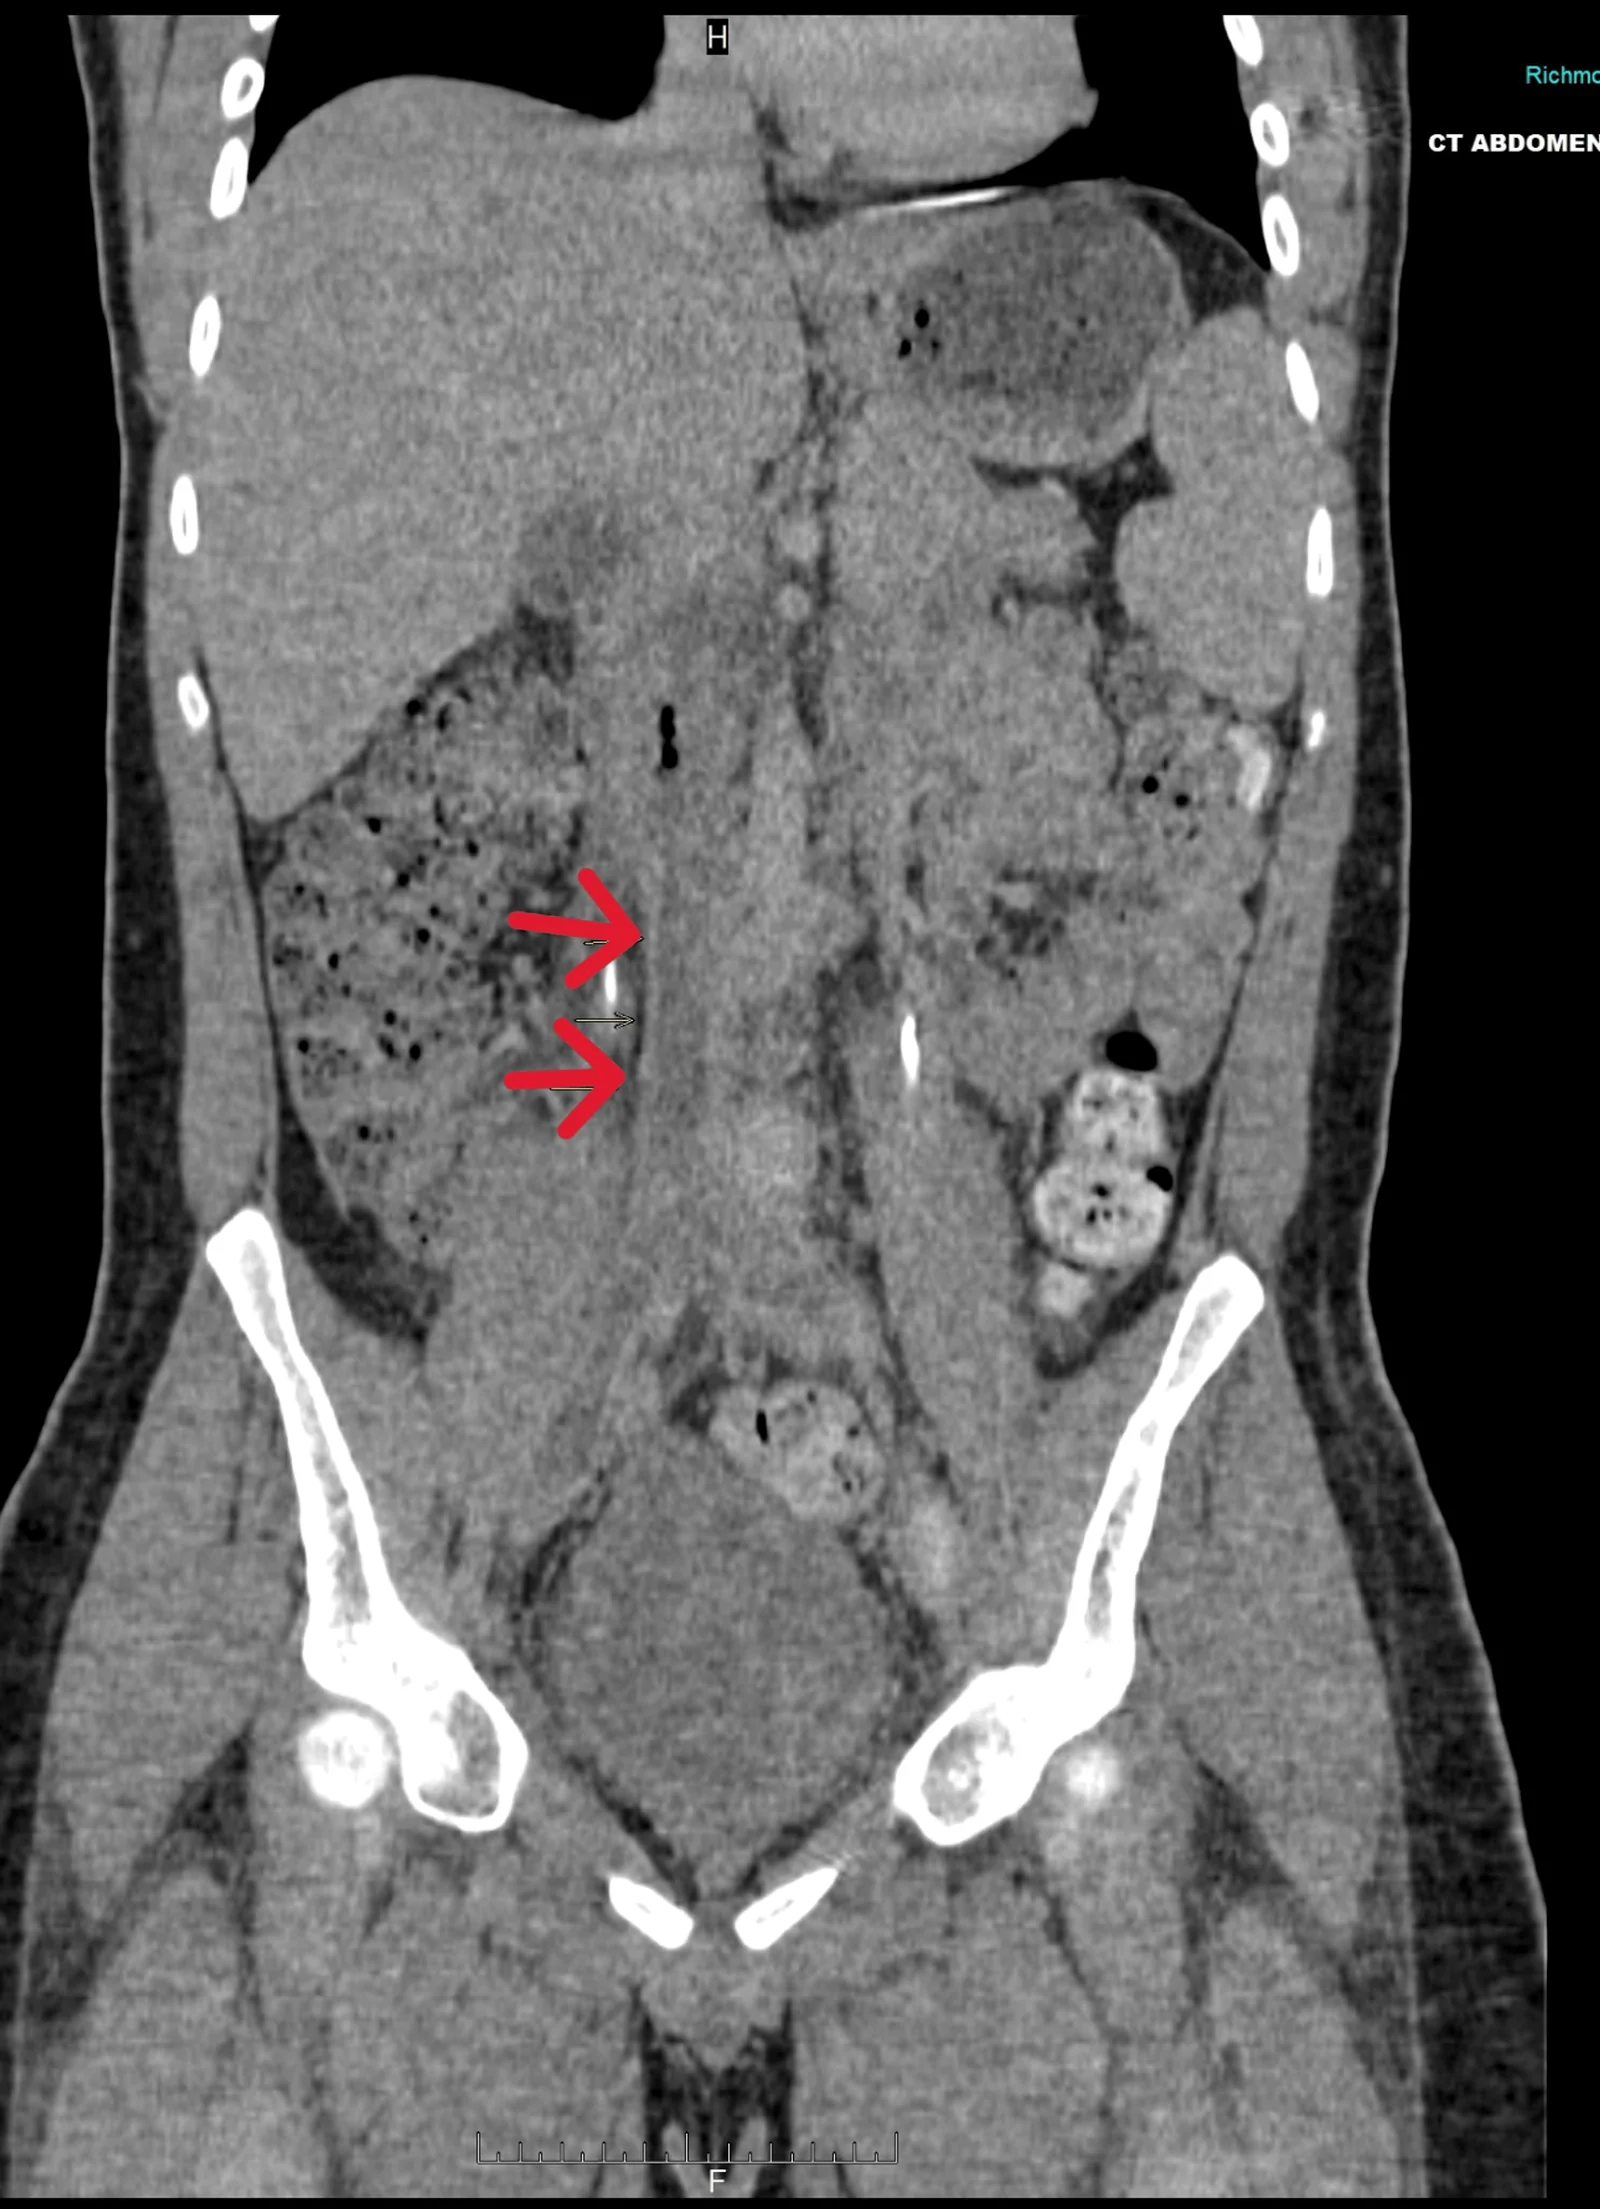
Coronal computed tomography angiography showing thrombus extending within the inferior vena cava (red arrows)
